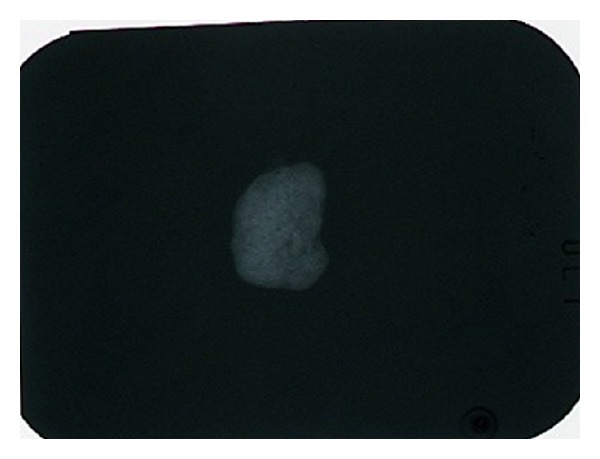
Dental X-rays showed an image of patchy calcification in the mass.
